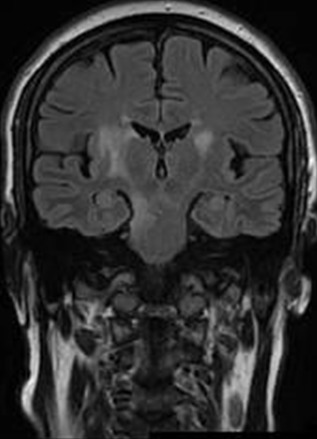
Coupe coronale en séquence FLAIR: anomalie de signal de la substance blanche para-ventriculaire bilatérale et latéro-protubérabtiel droite à type d'hyper signal
